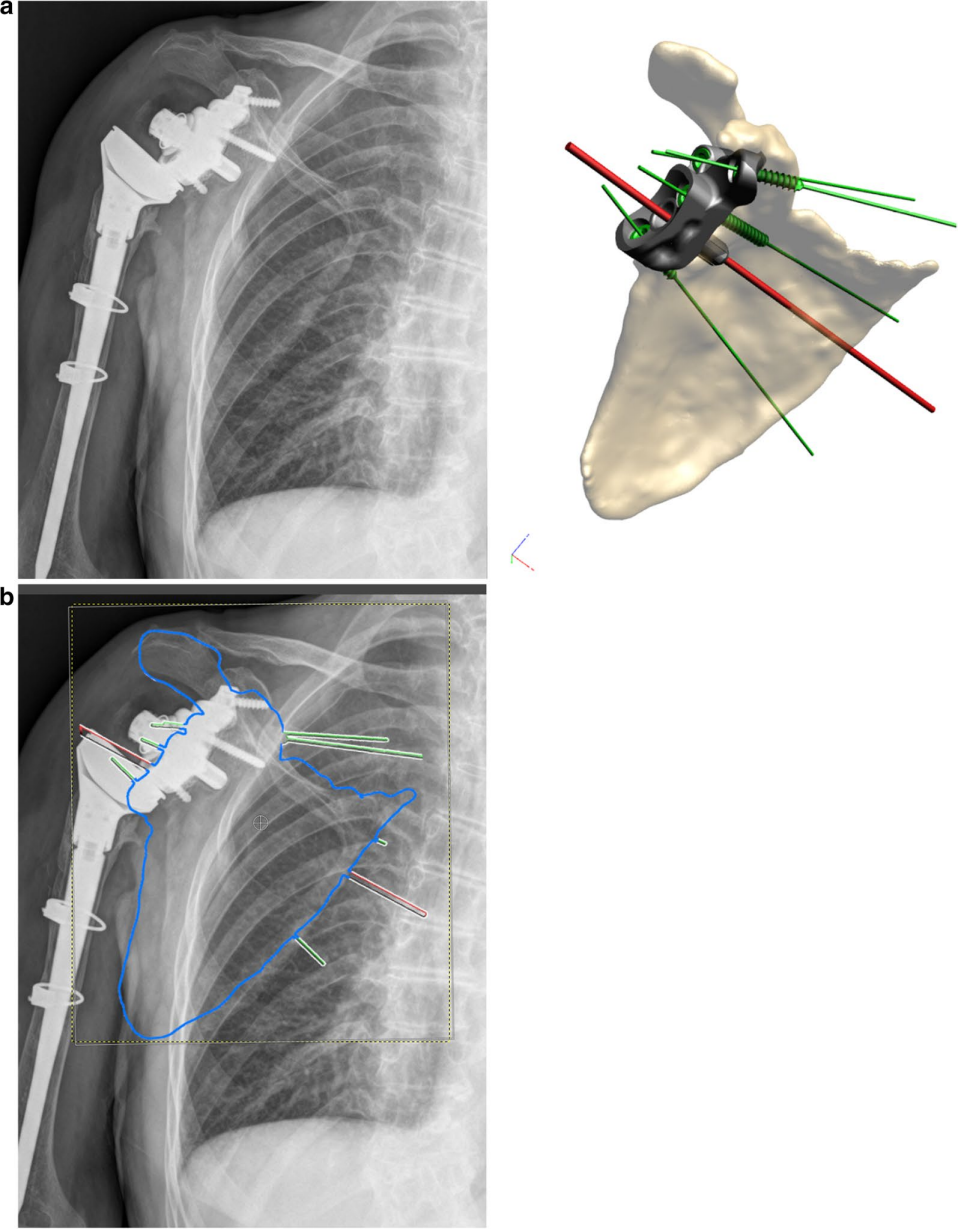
a Comparison between postoperative X-rays and preoperative plan. b Matching between X-rays and preoperative plan with reconstruction of peg and screw directions
